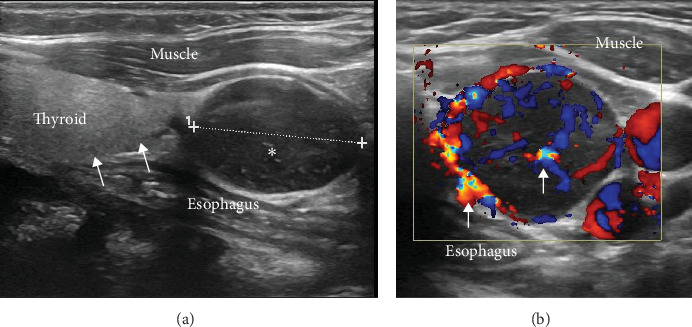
16-year-old girl with hyperparathyroidism. Longitudinal gray scale (A) and transverse color Doppler (B) ultrasound images of the left thyroid area. There is an oval well circumscribed 23 mm × 17 mm × 15 mm hypoechoic nodule (*⁣*^*∗*^ in subpart (A)) adjacent to the lower pole of the left thyroid lobe (arrows in subpart (A)). It shows increased central and peripheral vascularity on color Doppler (arrows in subpart (B)). Findings are consistent with a parathyroid adenoma.
